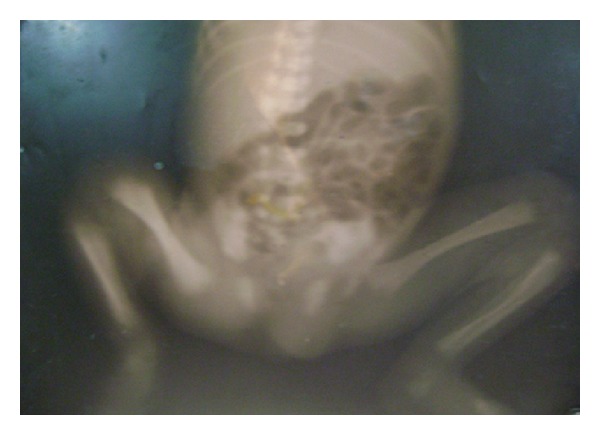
Radiograph of long bones: distal end of femur showing erosions.
